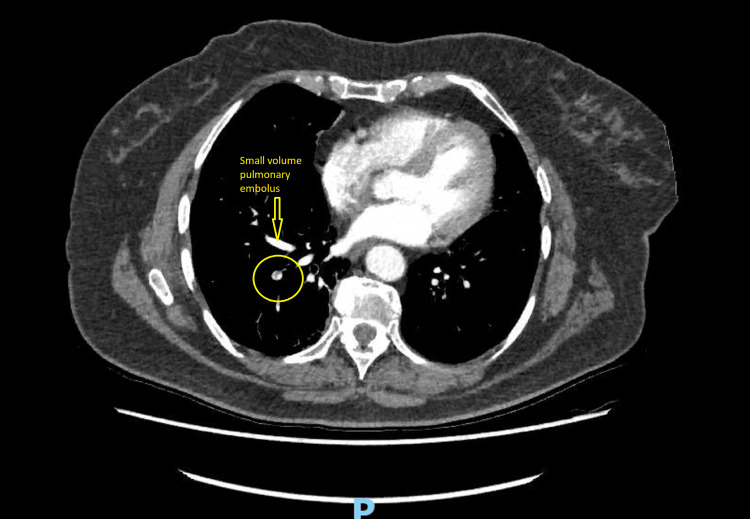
CT pulmonary angiogram CT pulmonary angiogram with small pulmonary embolus (CT was performed as a routine follow-up by the oncology team). CT: computed tomography
